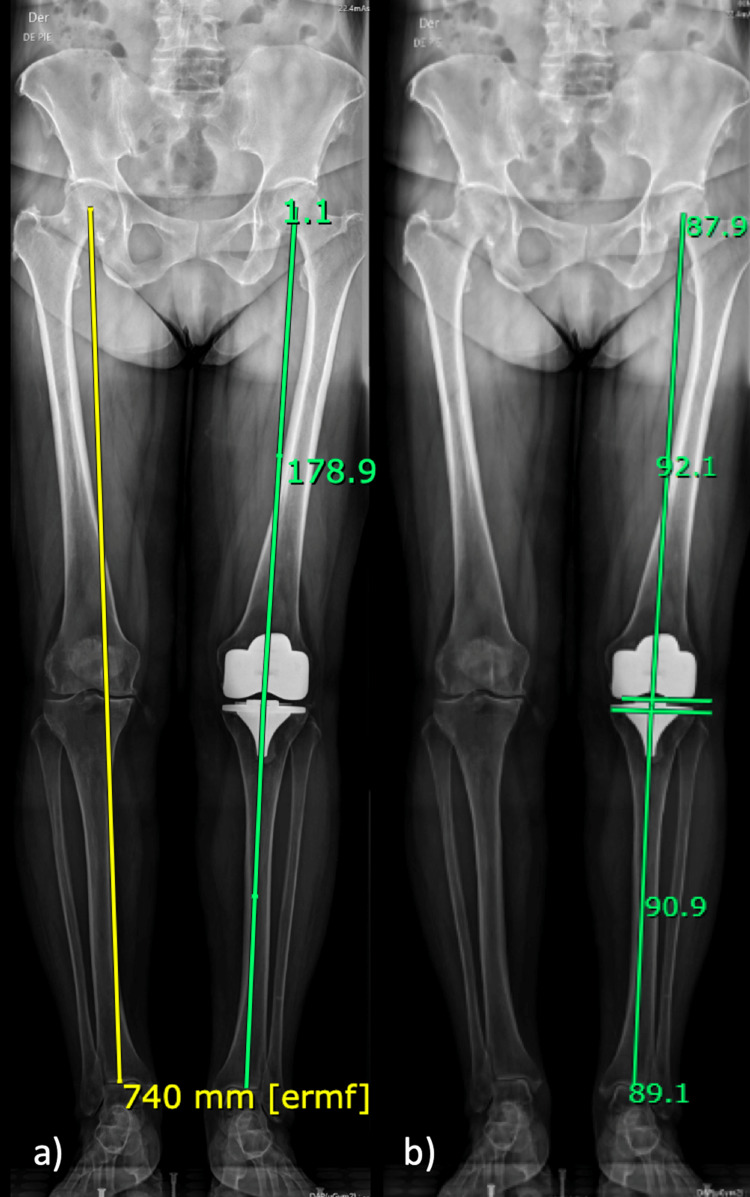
Full-length standing anteroposterior radiograph (a) Limb length (yellow line), the hip-knee-ankle angle (green line); (b) Mechanical lateral femoral component alignment, and mechanical medial tibial component alignment.
